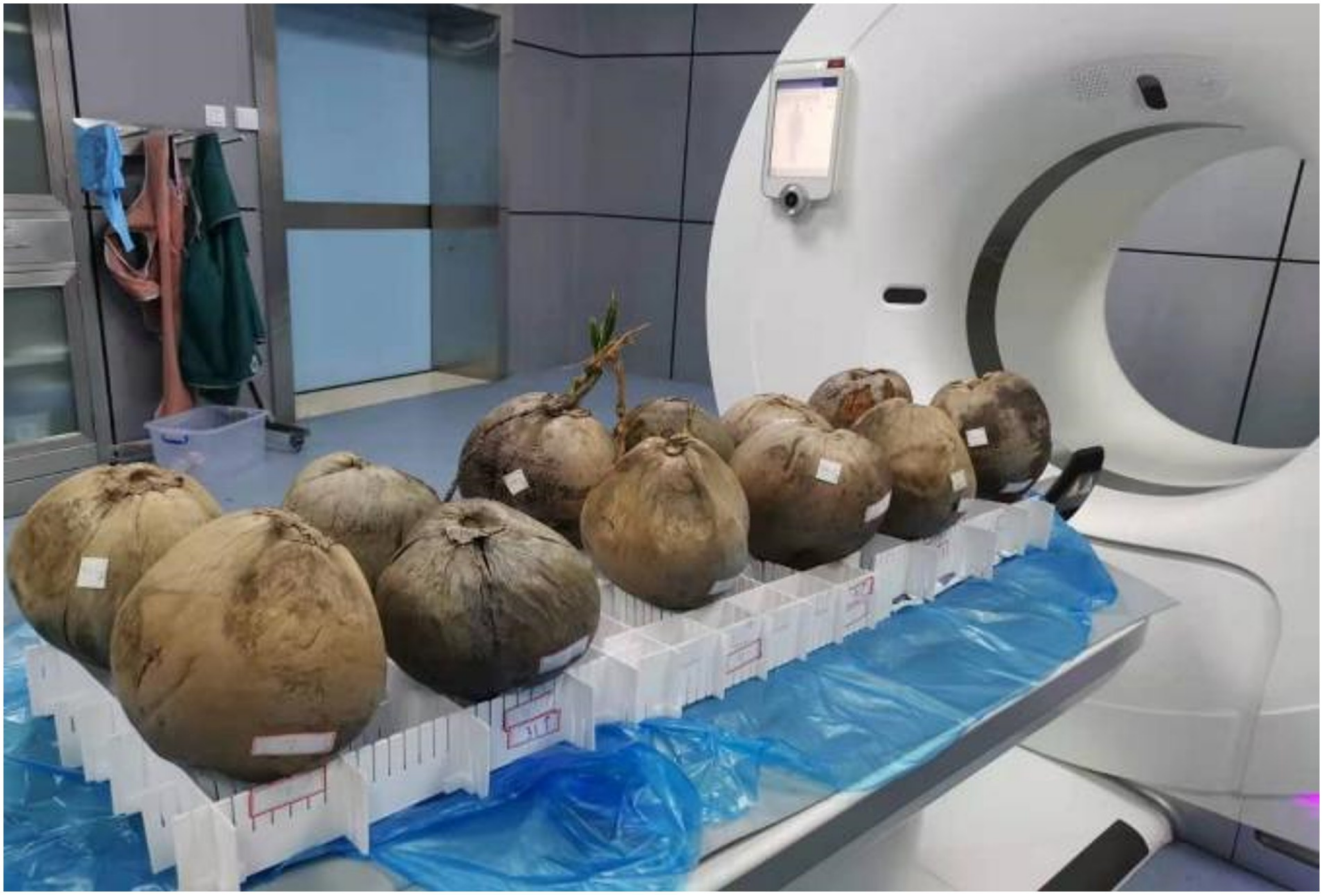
The position of the coconuts on the computed tomography scanner.
